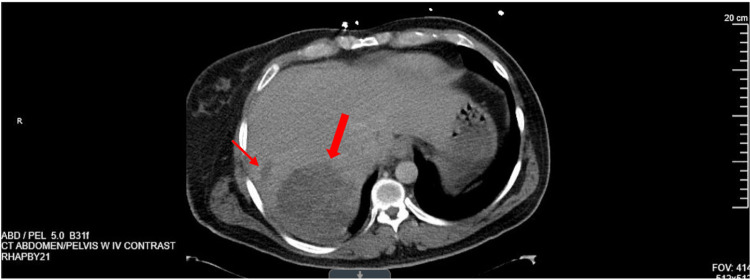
CT abdomen and pelvis without contrast demonstrating an indistinct hypodensity in the posterior segment of the right lobe, measuring 8.4 × 9.3 × 7.4 cm (large arrow), with an average density of 17 Hounsfield units, and a small ovoid hypodensity measuring 2.4 cm in the largest diameter (small arrow).
